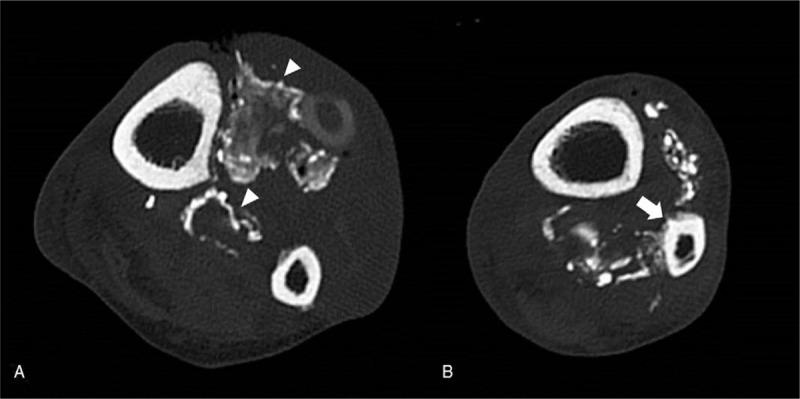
Axial computed tomography scan (A) showing a predominantly peripherally distributed calcified mass replacing the anterior and deep posterior compartment muscles of the leg (arrow head). Also, it (B) shows sclerotic changes and erosion of the medial and posterior cortices of the fibula (arrow).
